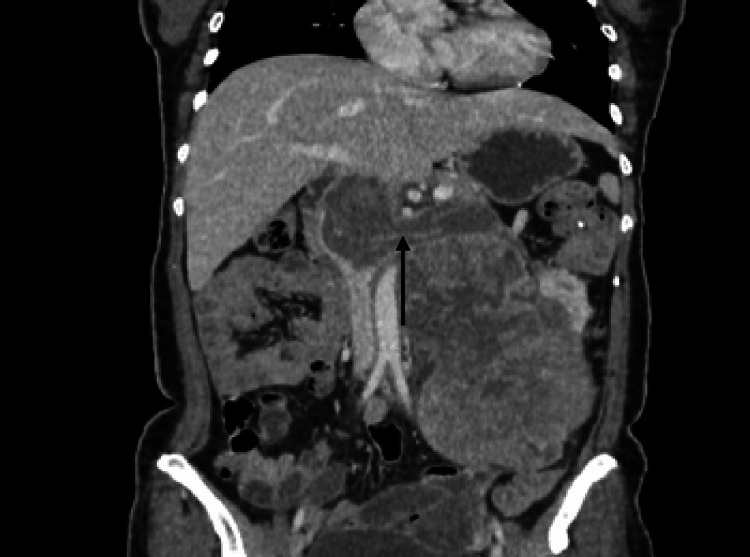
CECT abdomen coronal section showing extension of tumor thrombus from the left renal vein into the IVC. CECT: contrast-enhanced computed tomography; IVC: inferior vena cava
